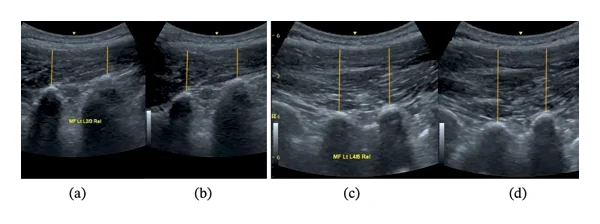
Ultrasound images of (a) L_2_–L_3_ relaxed multifidus muscle thickness, (b) L_2_–L_3_ contracted multifidus muscle thickness, (c) L_4_–L_5_ relaxed multifidus muscle thickness and (d) L_4_–L_5_ contracted multifidus muscle thickness.
